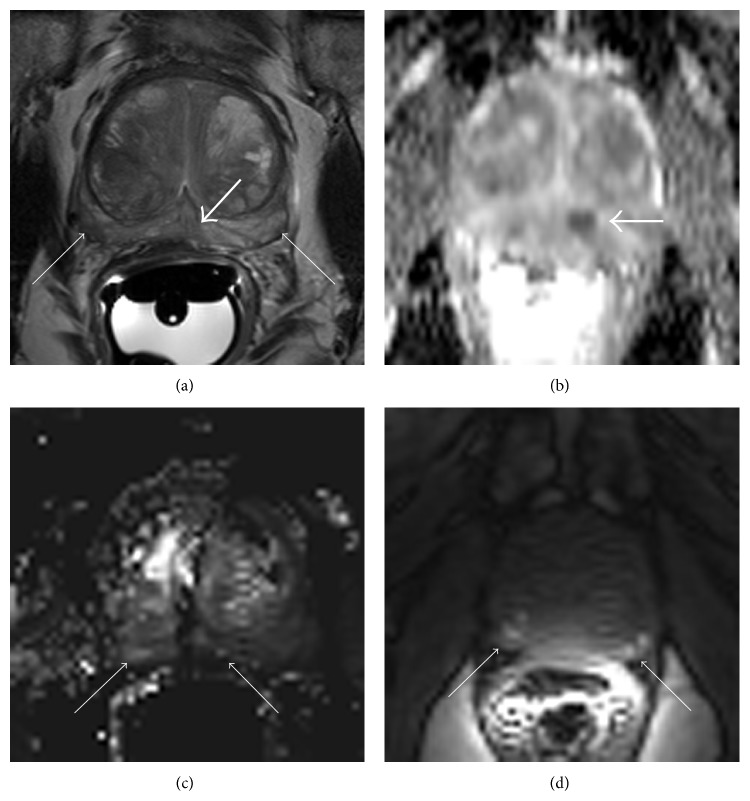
Hemorrhage can limit diagnostic confidence. The location of tumor (thick arrow) on T2-weighted imaging (a) is indistinct, with additional low signal areas in the peripheral gland bilaterally that appear mass-like (thin arrows). The location of tumor is confirmed on the apparent diffusion coefficient (ADC) map (b) but dynamic contrast-enhanced (DCE) perfusion (c) is heterogeneous (thin arrows). The precontrast T1-weighted image (d) reveals short T1 corresponding to the areas of low signal on T2-weighted imaging (thin arrows) confirming that this signifies artifact from hemorrhage.
